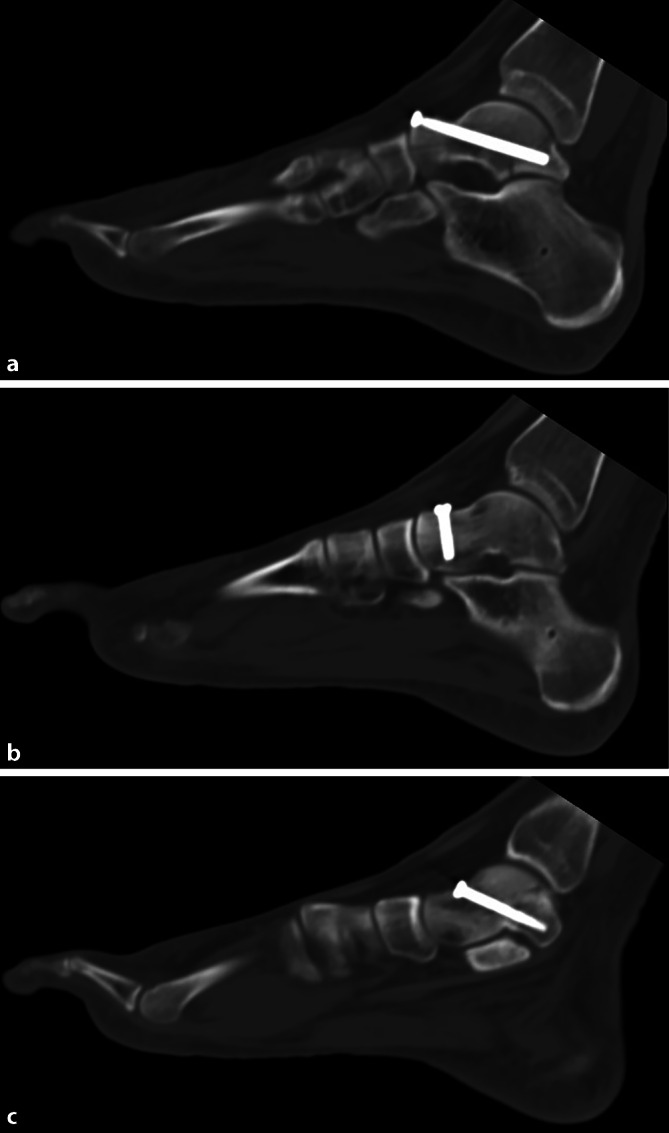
a–c Postoperative Kontroll-CT (a multiplanare Rekonstruktion sagittale Ebene Schraube 1; b multiplanare Rekonstruktion sagittale Ebene Schraube 2; c multiplanare Rekonstruktion sagittale Ebene Schraube 3)
